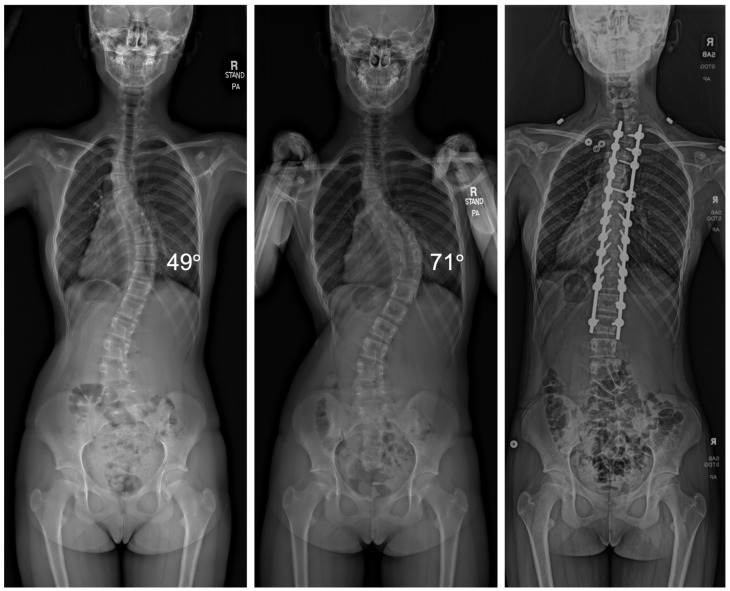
Radiographic imaging of a patient before and after a 6-month surgical delay that resulted in curve progression from 49° to 71°, requiring the transition from VBT to PSF.
